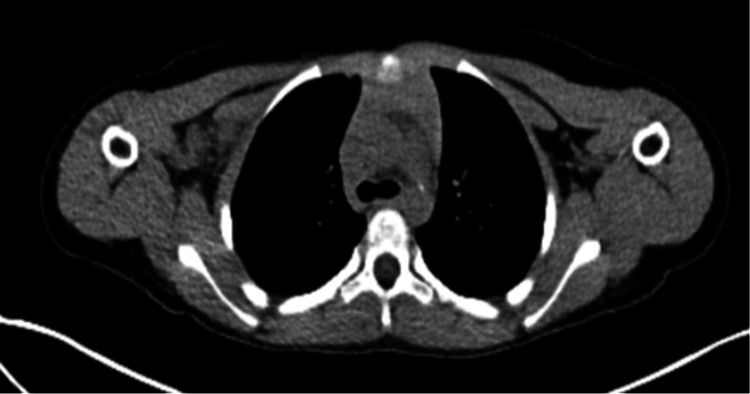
PET CT full-body scan demonstrating right axillary adenopathy and neoplastic uptake in the right lung.
